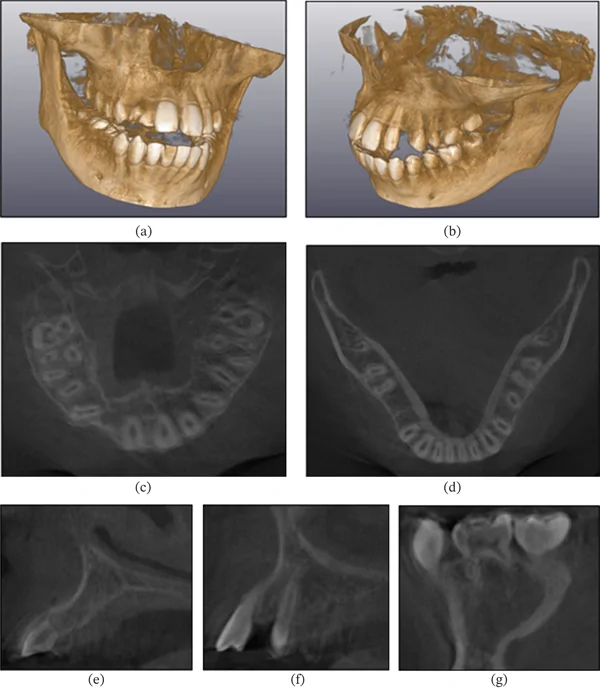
CBCT images. (a, b) Three‐dimensional reconstructions. (c, e) Right maxilla demonstrating absence of tooth germs for FDI 12 and 13. (f) Left maxilla demonstrating absence of the tooth germ for FDI 23. (d, g) Right mandible demonstrating absence of the tooth germ for FDI 45. No impacted or ectopically positioned teeth are visible in the corresponding regions.
